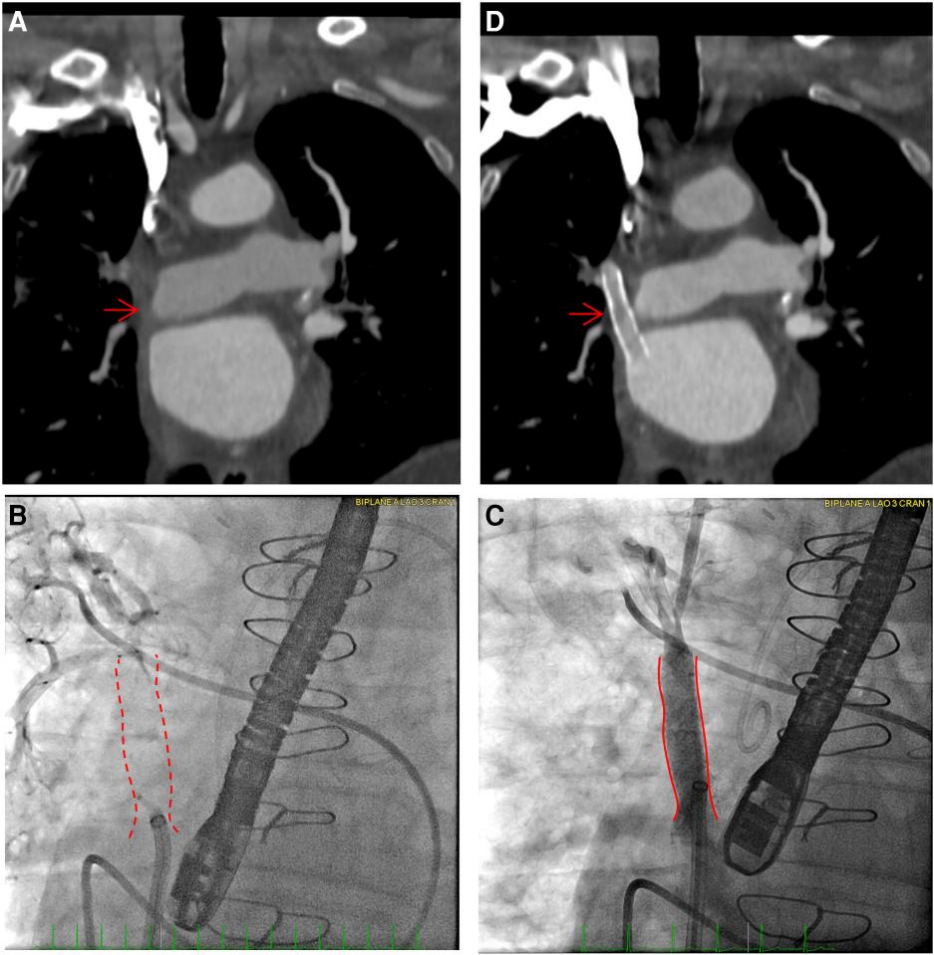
Case 3. (*A*) Computed tomography scan at 16 months post-surgery with obstructed baffle (red arrow). (*B*) Fluoroscopy showing obstructed baffle (red dotted lines). (*C*) Post-procedure fluoroscopy showing patent baffle (red solid lines). (*D*) Computed tomography scan performed 30 months post-stenting showing patent baffle (red arrow).
